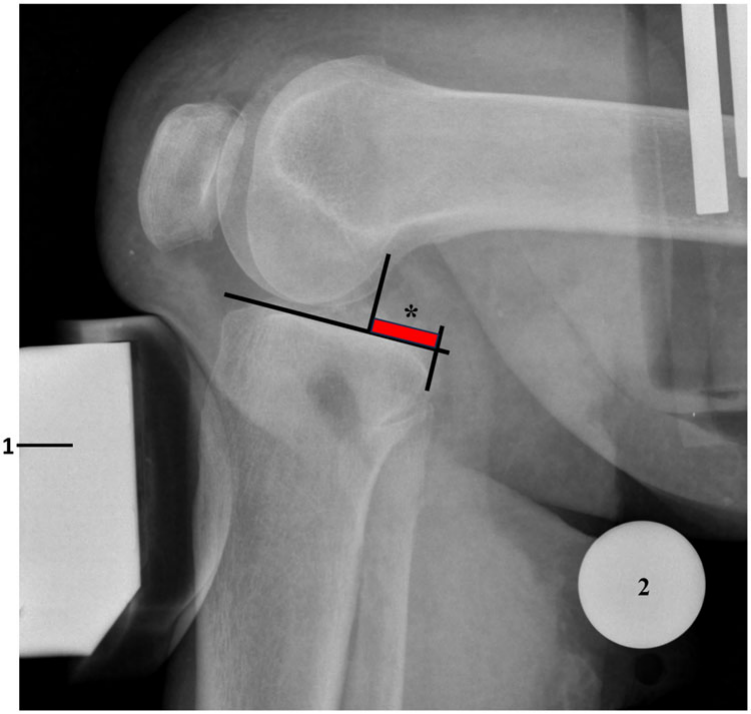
Radiographic posterior tibial displacement (PTD) according to Jacobsen [11] (1 = Pressure device of the Telos device; 2 = X‐ray reference ball with a diameter of 32 mm; *= PTD according to Jacobsen [11], which is the distance in between the two perpendicular lines).
